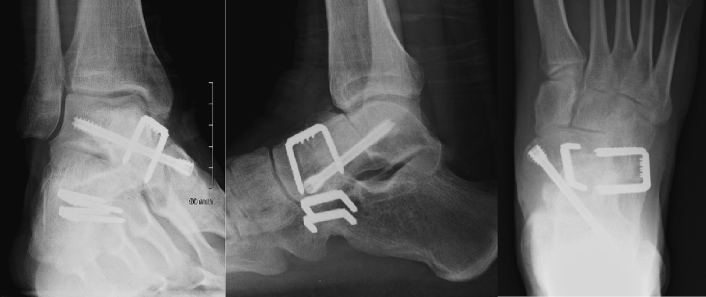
(A, B, C): Radiographs at 6 weeks post en bloc resection of the talar chondroblastoma. This radiograph demonstrates en bloc resection, femoral head allograft reconstruction of the talus and screw/staple fusion of the talonavicular and calcaneocuboid joints.
